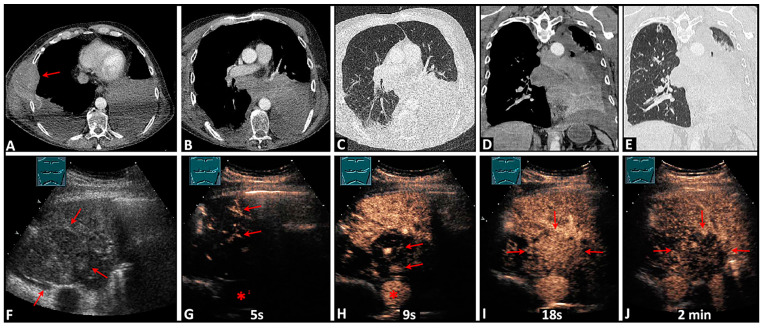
A 57-year-old patient with a bronchoscopically confirmed small cell bronchogenic carcinoma of the left lower lobe and contralateral rib and chest wall metastases (arrow) (A). On computed tomography scans (B–E), an obstructive atelectasis with pleural effusion is visible, but a tumor formation cannot be differentiated. On B-mode lung ultrasound (F), a central tumor is distinguishable from the downstream atelectasis (arrows). On contrast-enhanced ultrasound, the atelectatic tissue shows homogeneous early pulmonary arterial enhancement (arrows) after 5 s, before the aorta (*) (G). The tumor tissue exhibits homogeneous delayed bronchial arterial enhancement (arrows) after 9 s, simultaneously with the aorta (*), which is a sign of systemic vascularization (H). The tumor displays isoenhancement (arrows) compared with the atelectasis after 18 s (I), with rapid washout (arrows) after 2 min (J).
